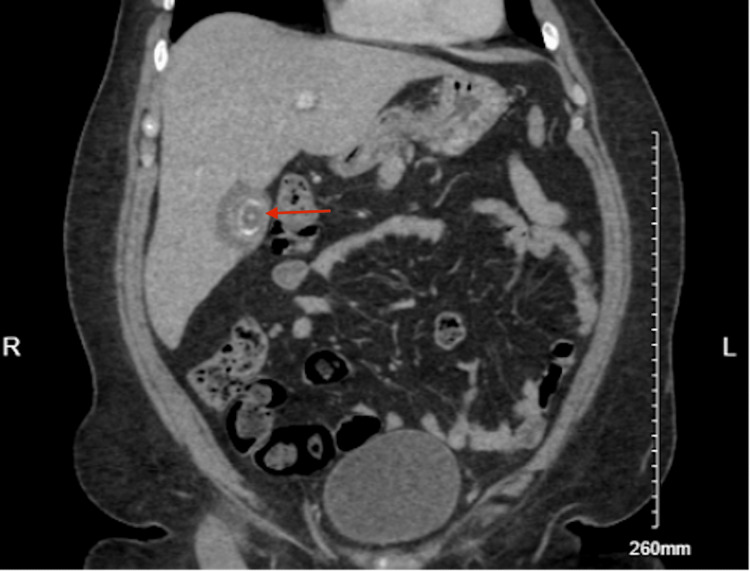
Abdomino-pelvic contrast CT-scan showed a mild degree of fluid around an under-distended gallbladder
